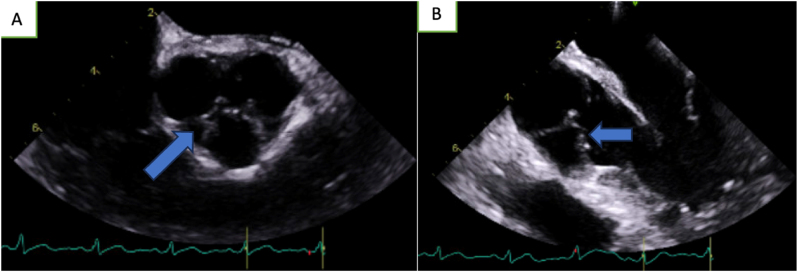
Transesophageal echocardiogram (TEE); (A and B) revealed no masses or vegetation seen in this study, there is no subaortic membrane, but there is a false tendon in the left ventricle (LV) close to the left ventricular outflow tract (LVOT) without hemodynamic effect.
